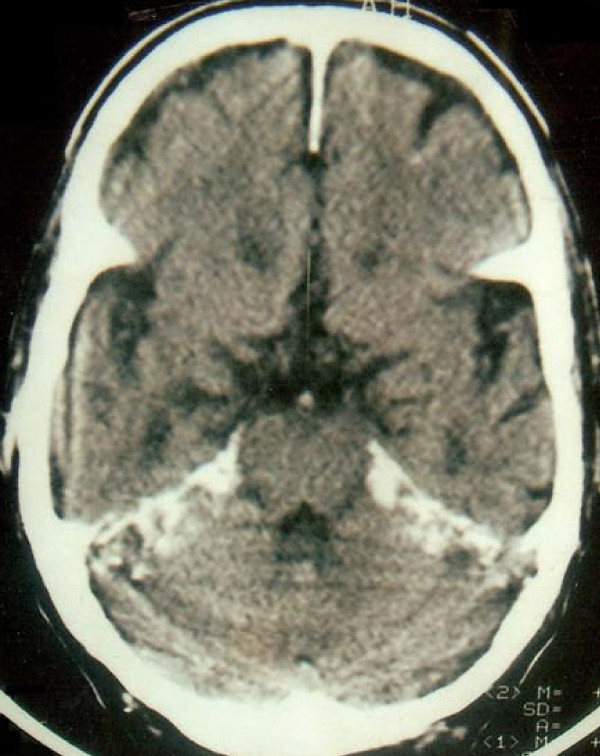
Computed tomography scan of the head, revealing diffuse, dense calcification of the cerebellum, tentorium, and falx.
